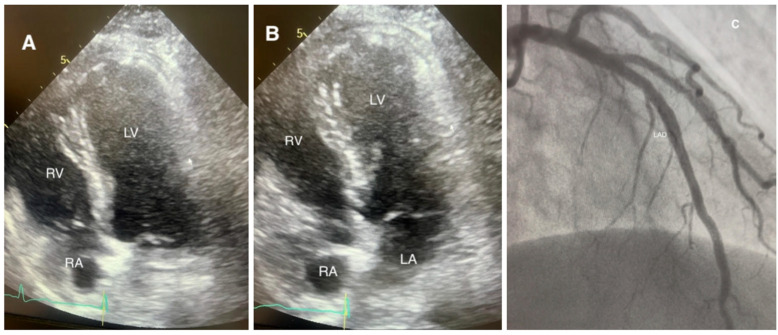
Apical four-chamber echocardiographic views of a female patient with Takotsubo syndrome following an incident of domestic abuse, admitted with chest pain and elevated cardiac enzymes. (A) Left ventricle during diastole. (B) Left ventricle during systole with akinetic and ballooned LV apex. (C) Coronary angiogram demonstrating patent coronary arteries. RV = right ventricle; RA = right atrium; LV = left ventricle; LA = left atrium; LAD = left anterior descending artery. (Courtesy of Dr. Raed Bargout).
